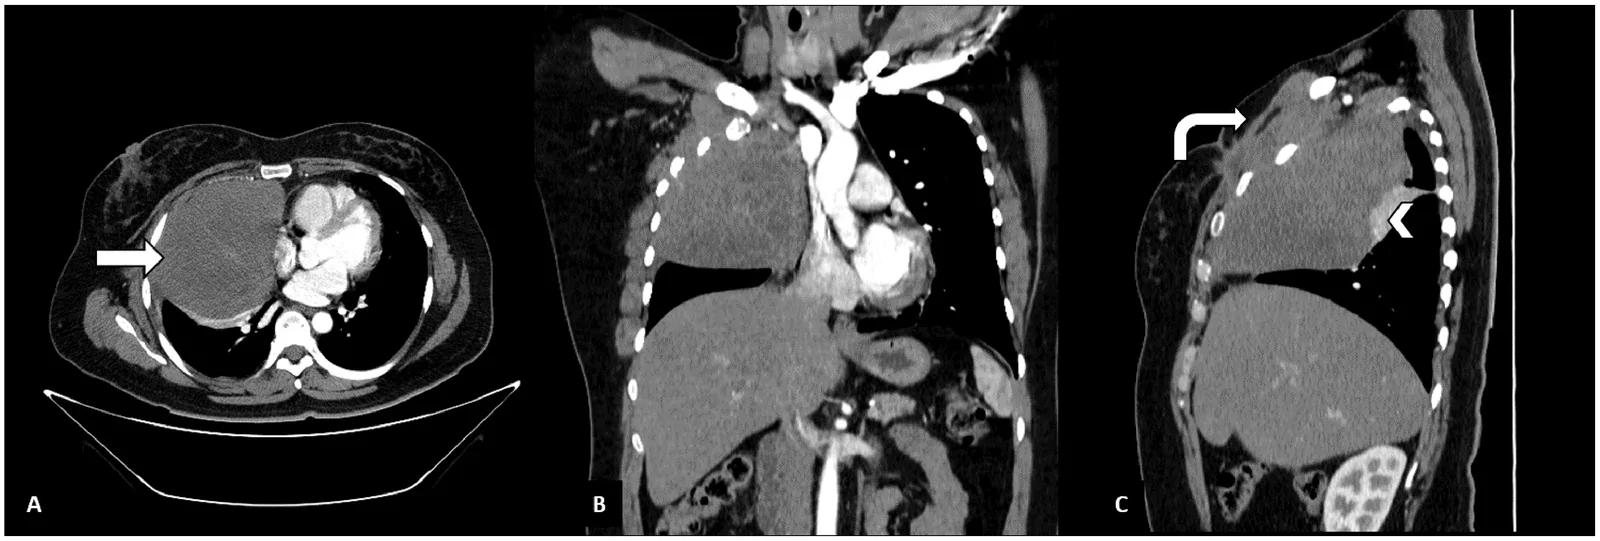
Arterial-phase contrast-enhanced chest CT showing a large right pleural-based mass. (A) Axial image demonstrates a well-defined heterogeneous mass measuring approximately 14 × 9 × 15 cm (arrow), with partial right upper and middle lobe atelectasis and leftward cardiac displacement. (B) Coronal reconstruction shows its craniocaudal extent and mass effect. (C) Sagittal reconstruction demonstrates extension into the anterior chest wall and infraclavicular soft tissues (curved arrow) and compressive atelectasis (arrowhead).
